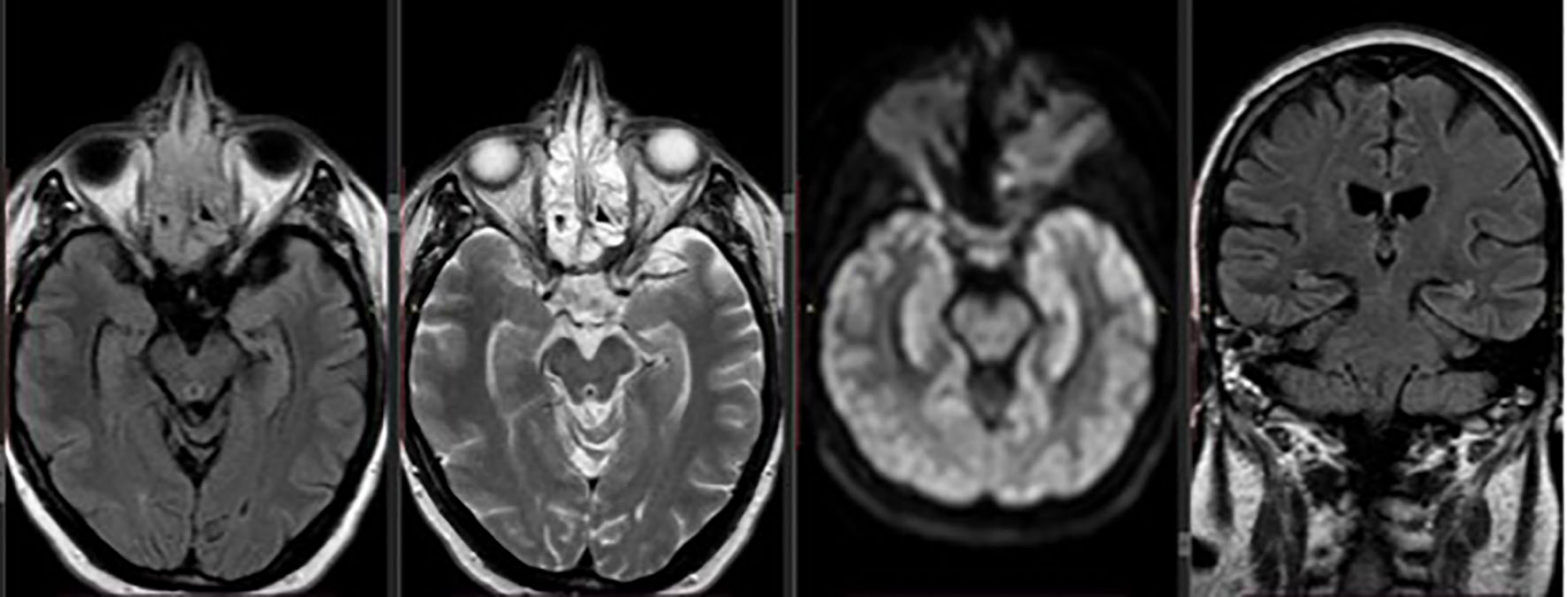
Axial and coronal follow-up brain MRI showing complete resolution of the previously noted periaqueductal hyperintensities, with no remaining signs of encephalopathy.
